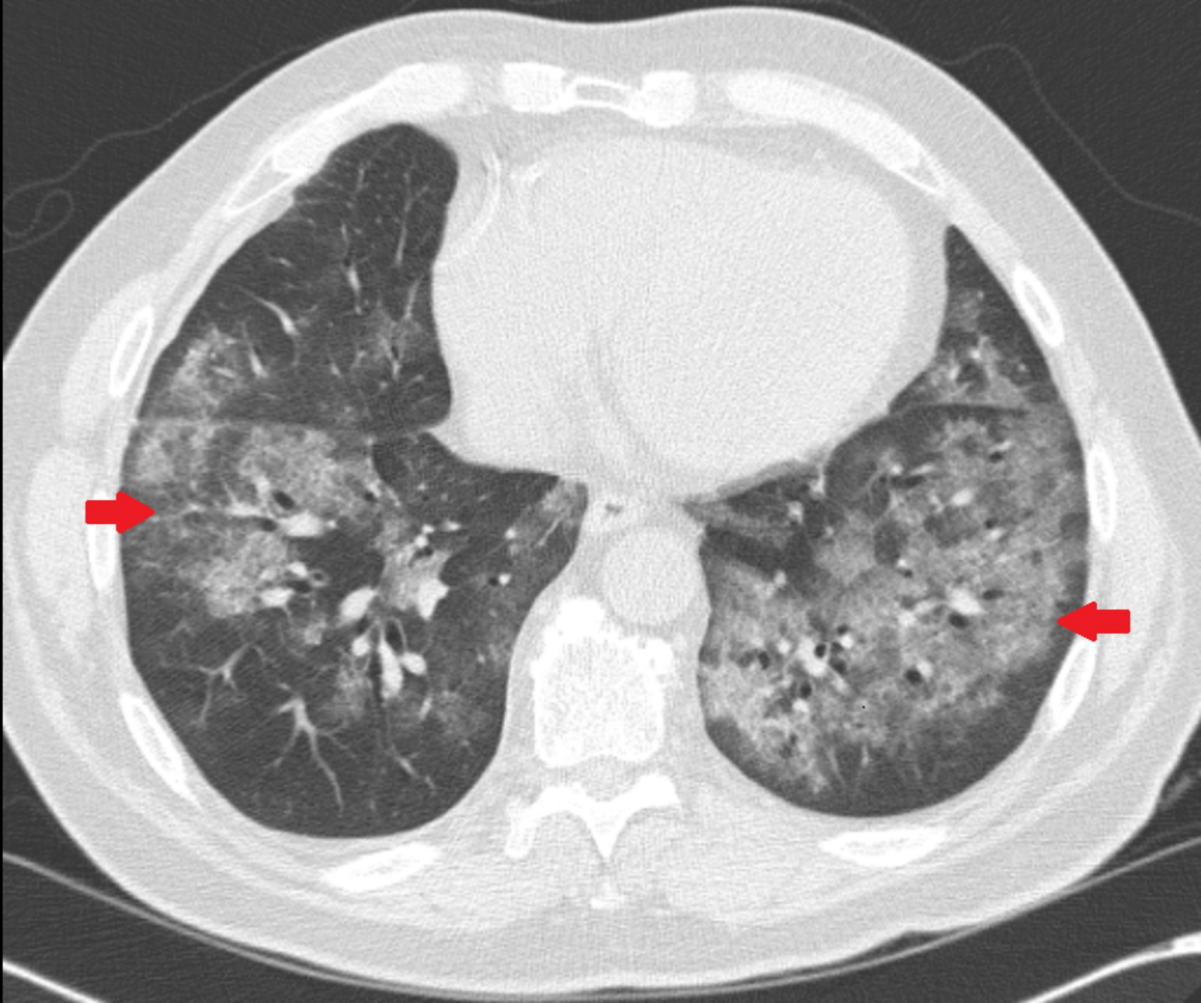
Axial CT chest image showing bilateral diffuse opacities (arrows)
